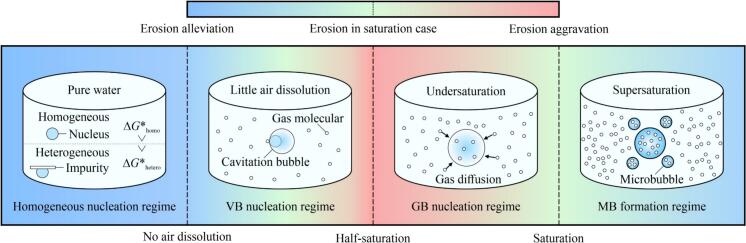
Conceptual classification of regimes influencing ultrasonic cavitation erosion in aqueous solutions containing dissolved air. Darker red and blue shades represent increasing aggravation and alleviation of cavitation erosion, respectively. Green shade represents minimal change in mass loss compared to the saturation condition. Δ*G_homo_ and Δ*G_hetero_ represent the free energy barriers for homogeneous and heterogeneous nucleation, respectively. (For interpretation of the references to colour in this figure legend, the reader is referred to the web version of this article.)
